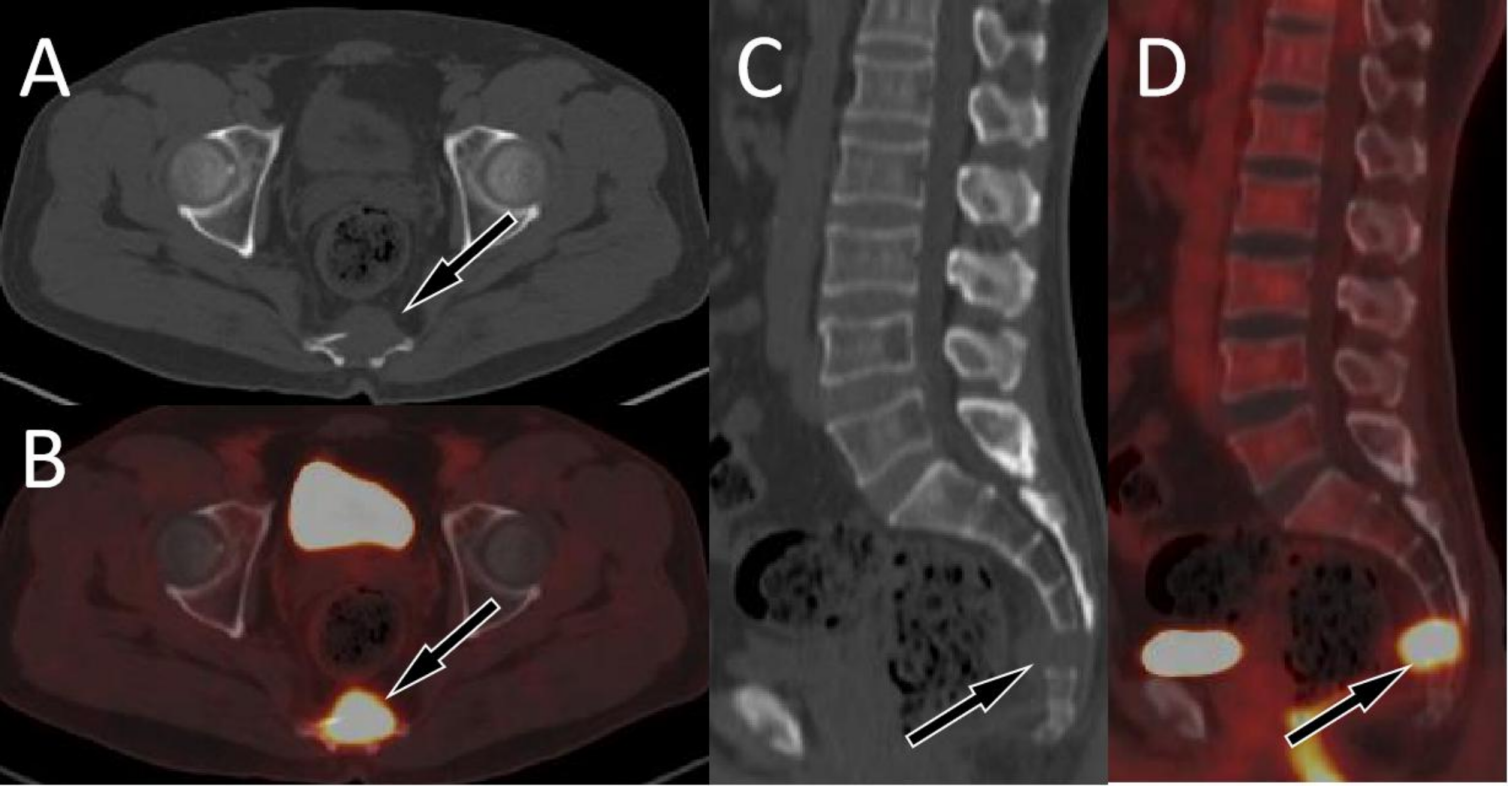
A 47-year-old woman with typical PET/CT findings of GCTB; (A) Axial CT shows osteolytic bone destruction in the sacral vertebrae, with slightly low-density soft tissue mass formation (arrow); (B) The PET/CT fusion image at the same level shows significantly increased ^18^F-FDG uptake, with a SUVmax of 12.5; Sagittal (C CT; D PET/CT) shows almost complete dissolution of the affected sacral vertebrae (arrows).
